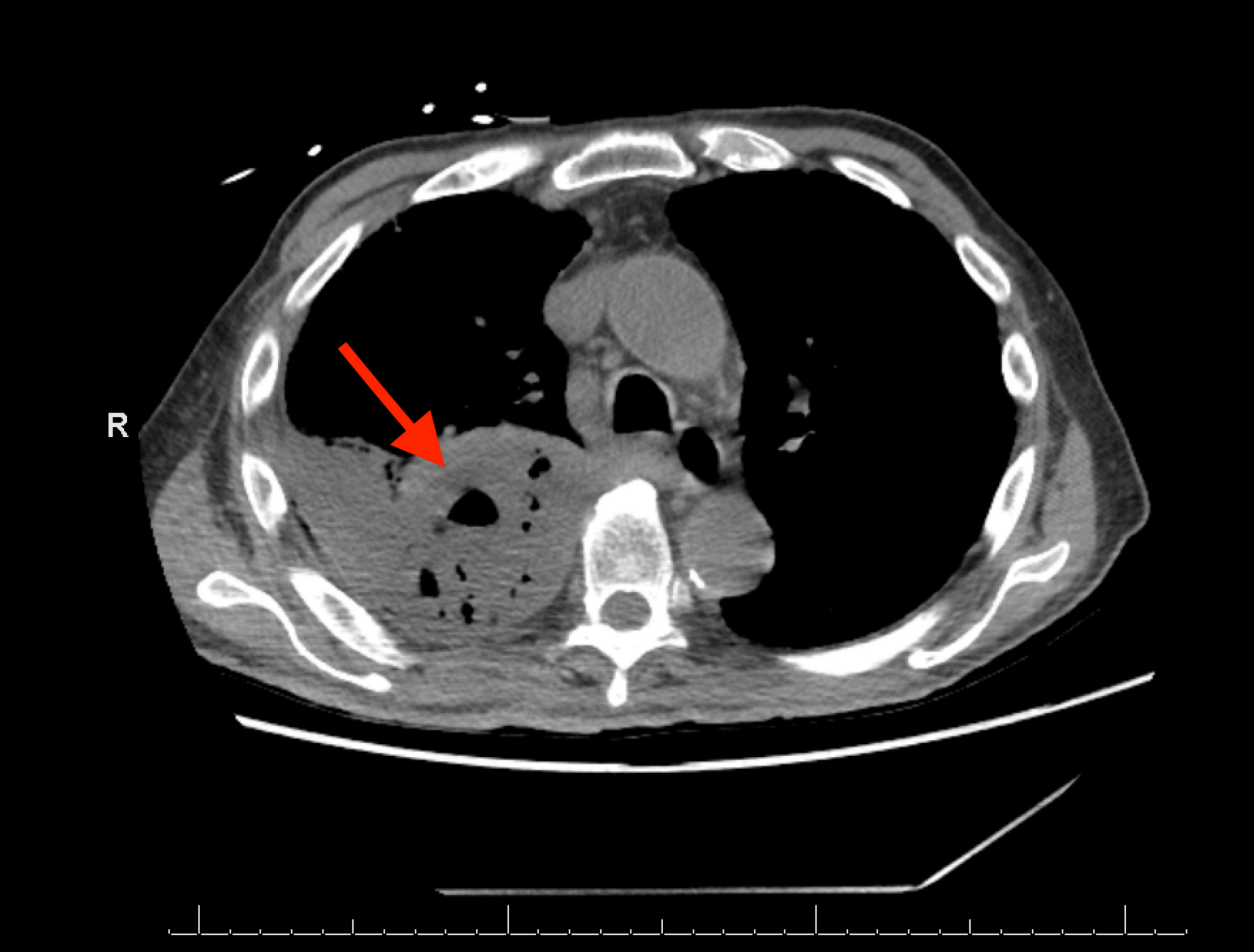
Chest CT without contrast demonstrating a right-sided pleural solid and a cystic gas lesion
